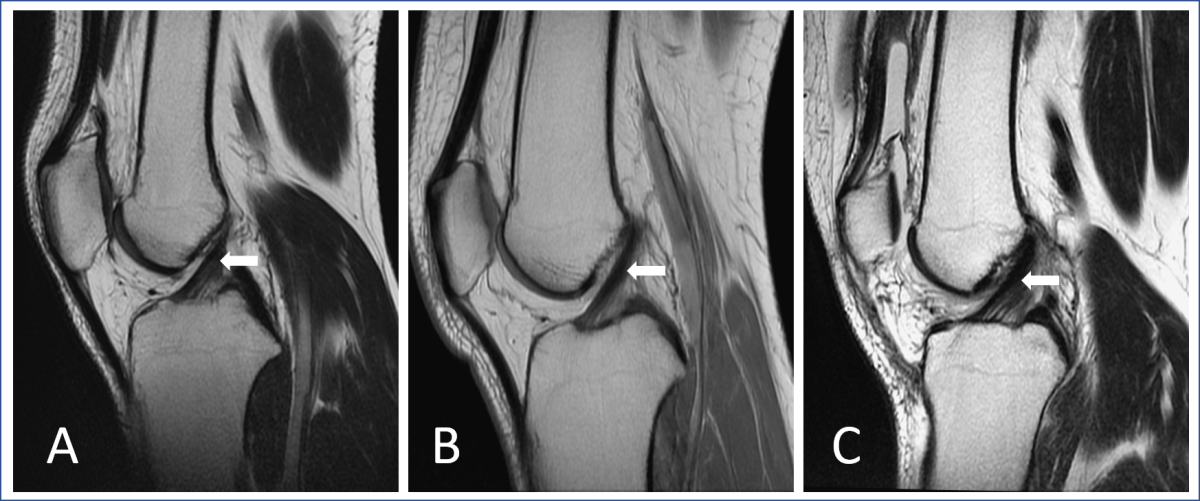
MR images of intact ACL. Sagittal proton density images of 3 different patients are shown. All images show the taut and straight bands parallel to the intercondylar roof with low signal intensity patterns of the intact ACL (white arrow). ACL: anterior cruciate ligament; MR: magnetic resonance.
